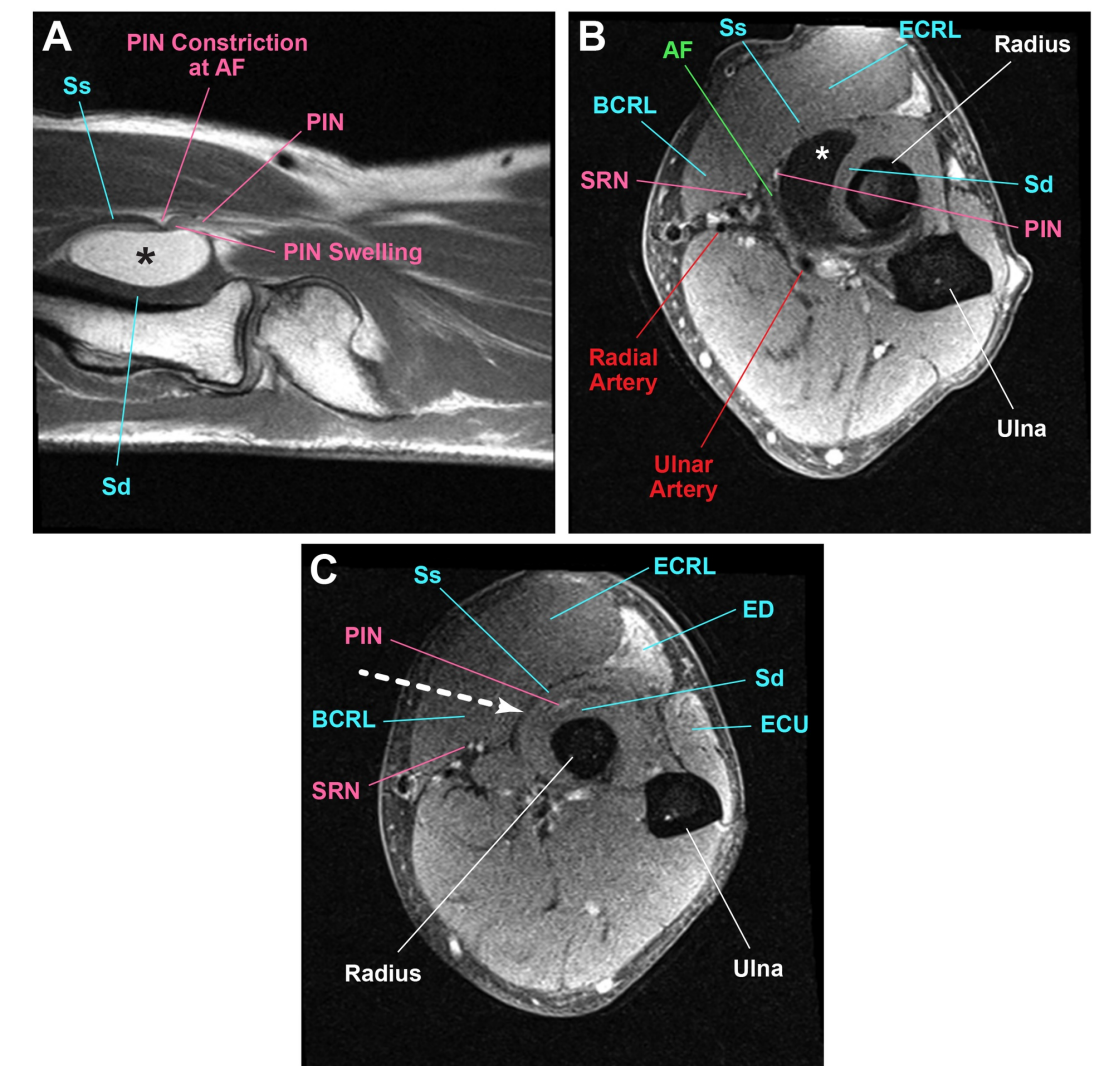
MRI showing a homogeneously hyperintense lesion (A) T1-weighted sagittal magnetic resonance imaging shows a hyperintense lipoma lesion (*) causing the swelling of the PIN proximal to the constriction at the AF. (B) T2-weighted axial shows the PIN entering between the AF and the lipoma. (C) T2-weighted axial further distally shows the PIN continuing distally between the superficial (Ss) and deep (Sd) heads of the supinator. The direction of approach between the BCRL and ECRL is shown (white arrow). Hyperintensity is seen in the ED and ECU. Abbreviations (a-z): Arcade of Frohse (AF), brachioradialis (BCRL), extensor carpi radialis longus (ECRL, extensor carpi ulnaris (ECU), extensor digitorum (ED), Lipoma (*), magnetic resonance imaging (MRI), posterior interosseous nerve (PIN), deep head of supinator (Sd), superficial radial nerve (SRN), superficial head of supinator (Ss)
